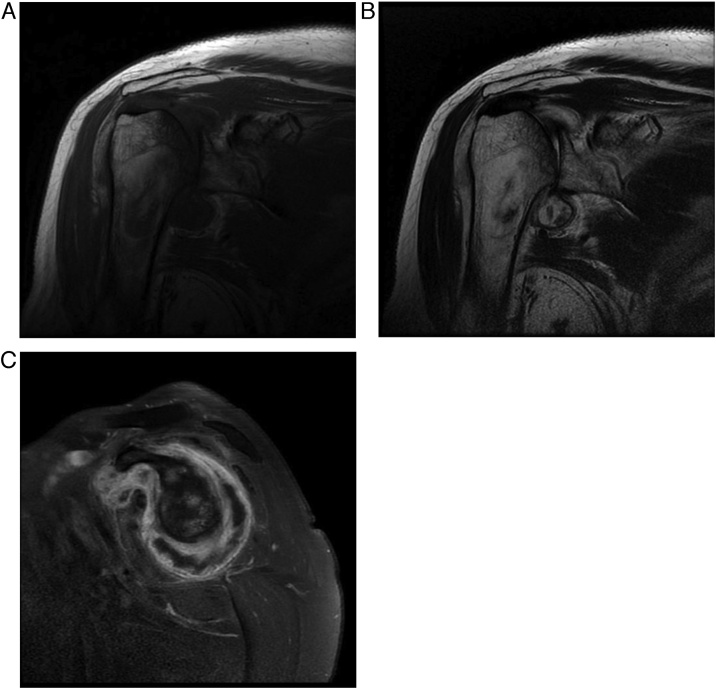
Right shoulder magnetic resonance image, T1-weighted image showing a low signal intensity mass-like lesion (a). T2-weighted image showing low signal change, suggesting presence of hemosiderin deposit (b). Enhanced view showing enhancement of the mass-like lesion (c).
